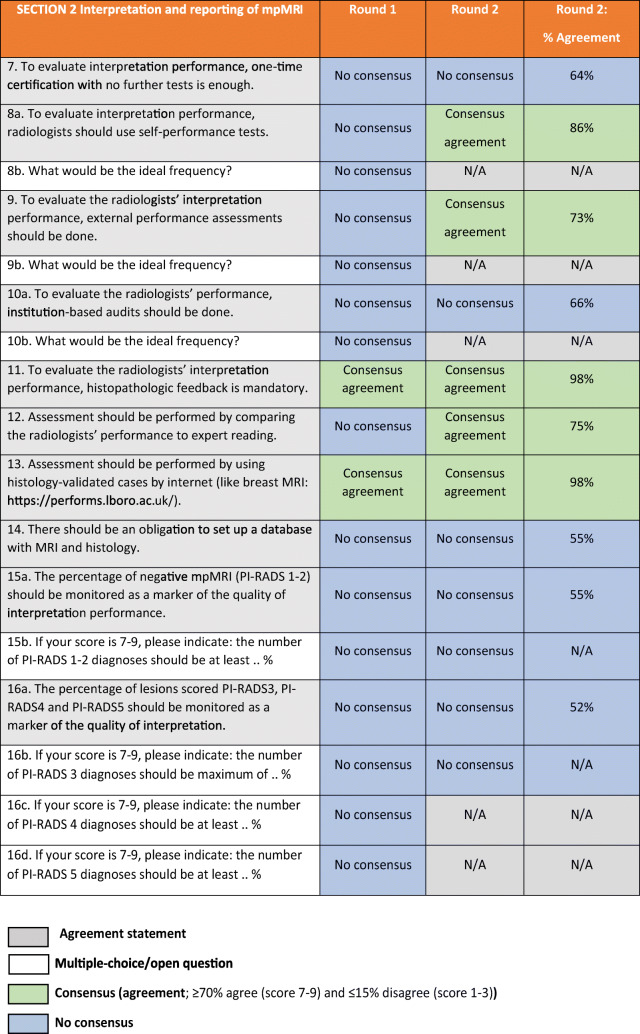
ESUR/ESUI consensus outcomes for section 2: Interpretation and reporting of mpMRI. *ADC* apparent diffusion coefficient, *ESUI* EAU Section of Urologic Imaging, *ESUR* European Society of Urogenital Radiology, *mpMRI* multi-parametric MRI, *N/A* not applicable
